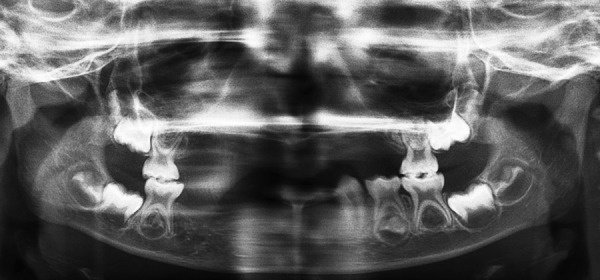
The panoramic radiograph revealed the presence of maxillary and mandibular right and left first permanent molar tooth buds, lower left first and second premolar tooth bud and lower right and left second permanent molar tooth buds. Also it reveals beginning of calcification of lower right second premolar
